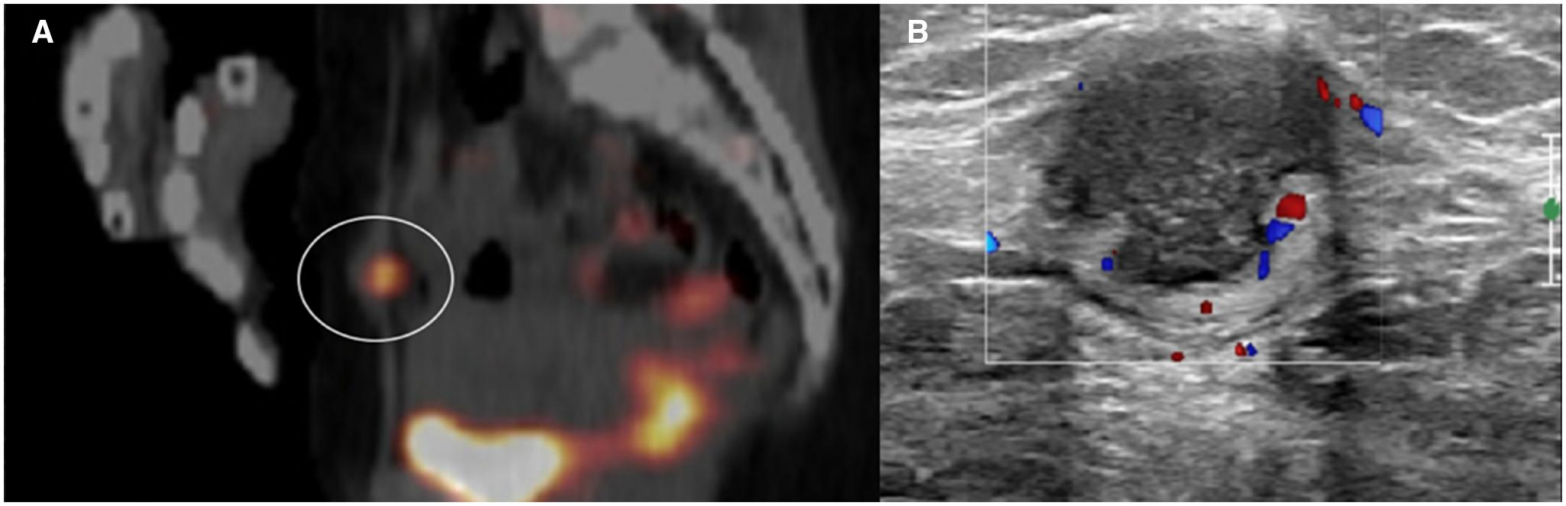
34-year-old female undergoing FDG PET/CT (A) for papillary thyroid carcinoma, which identified an FDG-avid subcutaneous anterior abdominal wall nodule (circled). (B) Further evaluation with US showed a non-tender, non-compressible lower abdominal wall nodule with irregular, hyperechoic margins and homogenous internal echoes with posterior acoustic enhancement. Mild peripheral vascularity is noted. This nodule was proven to be benign endometriosis on ultrasound-guided fine needle aspiration.
